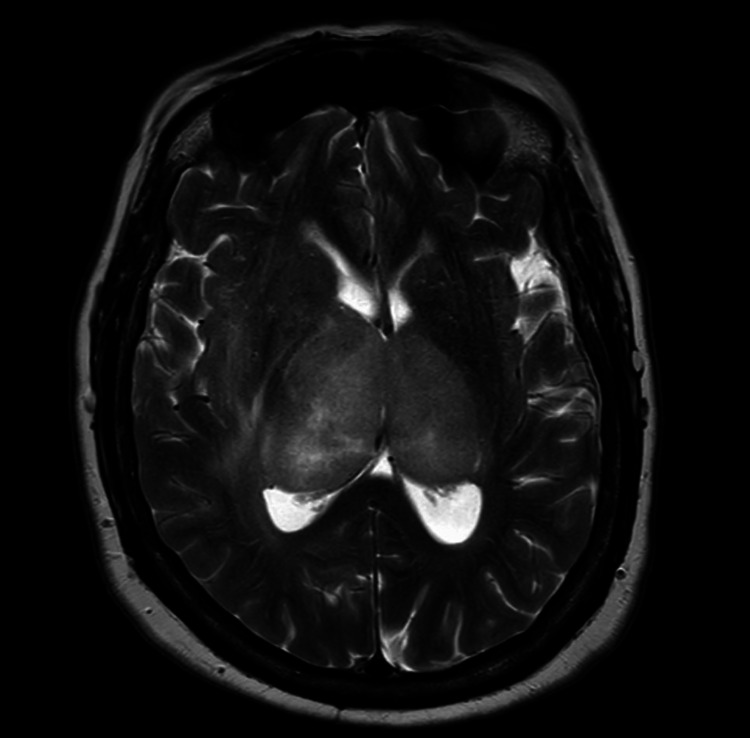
T2-weighted magnetic resonance image The image demonstrates heterogeneously T2 hyperintense lesions in the right greater than left thalami with associated elevated T2 signal in the right internal capsule and adjacent white matter.
